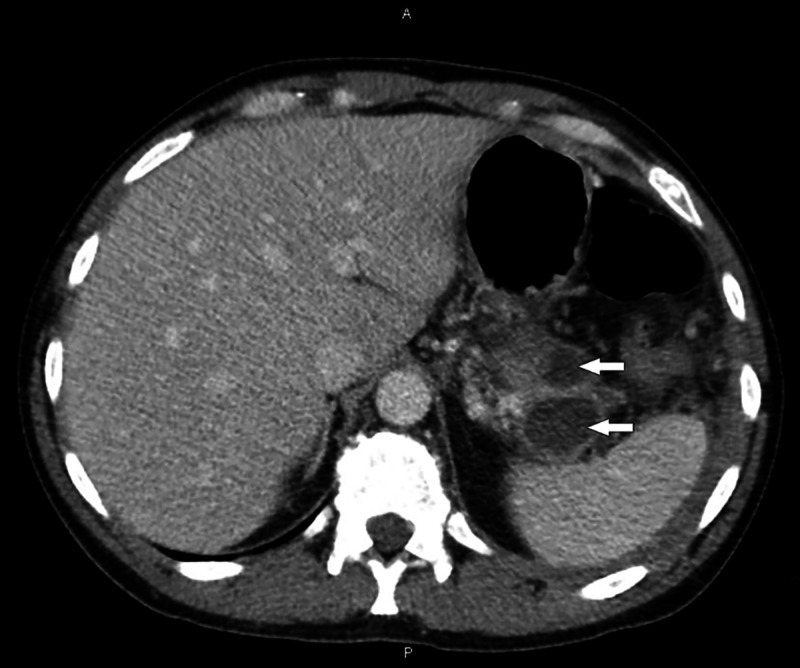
Previous CT scan of abdomen showing two pseudocysts in the pancreatic tail (white arrows).
